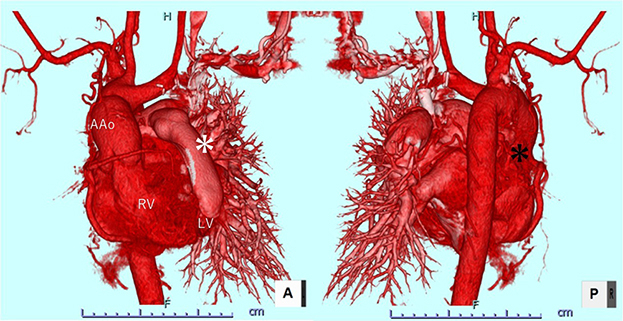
Postoperative contrast computed tomography image. *Black asterisk* indicates occluded right PA; *white asterisk* indicates LV-PA conduit.
